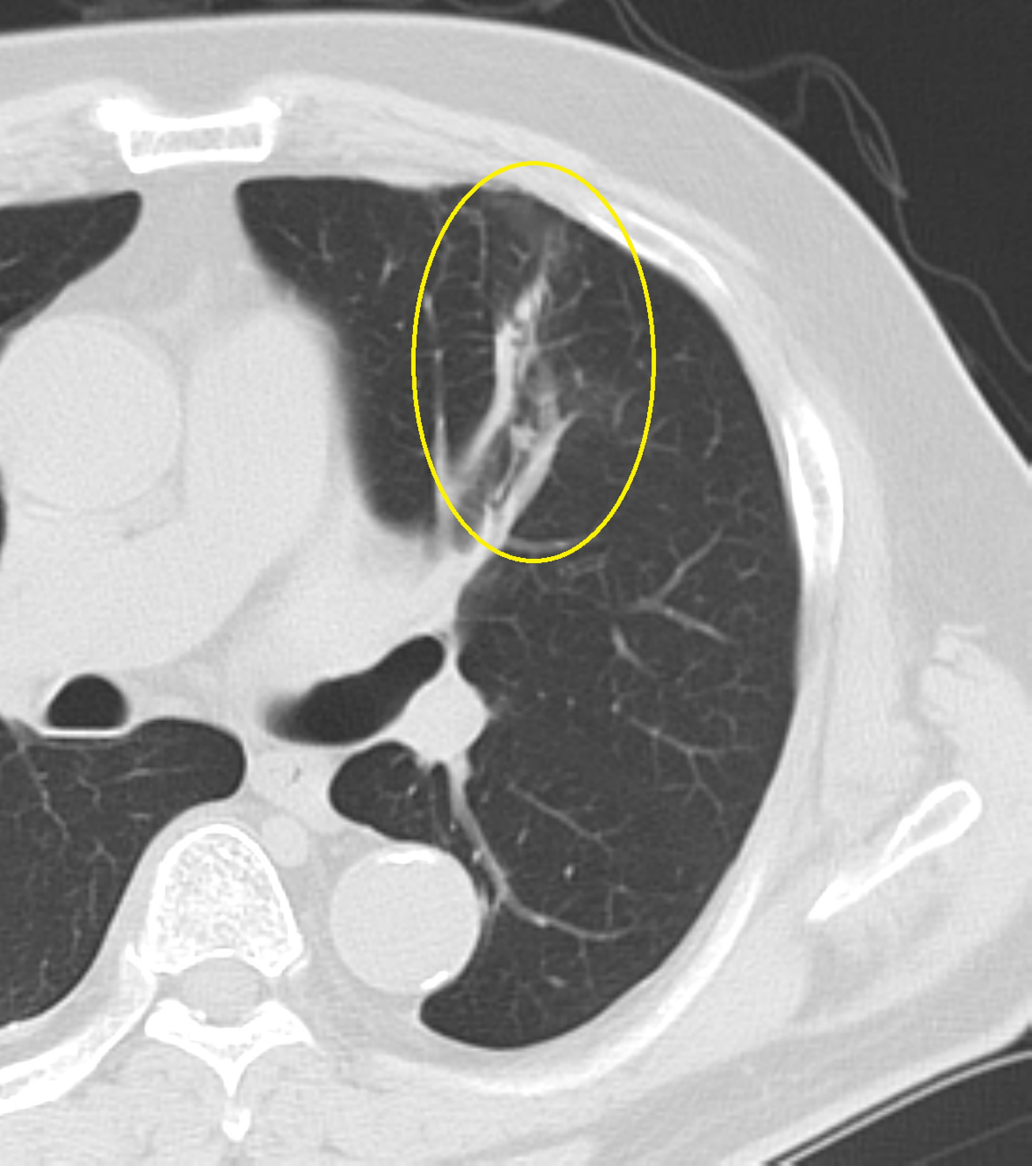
Chest plain CT taken one year later One year later, CT showed that the mass-like lesion became a cord-like shadow.
